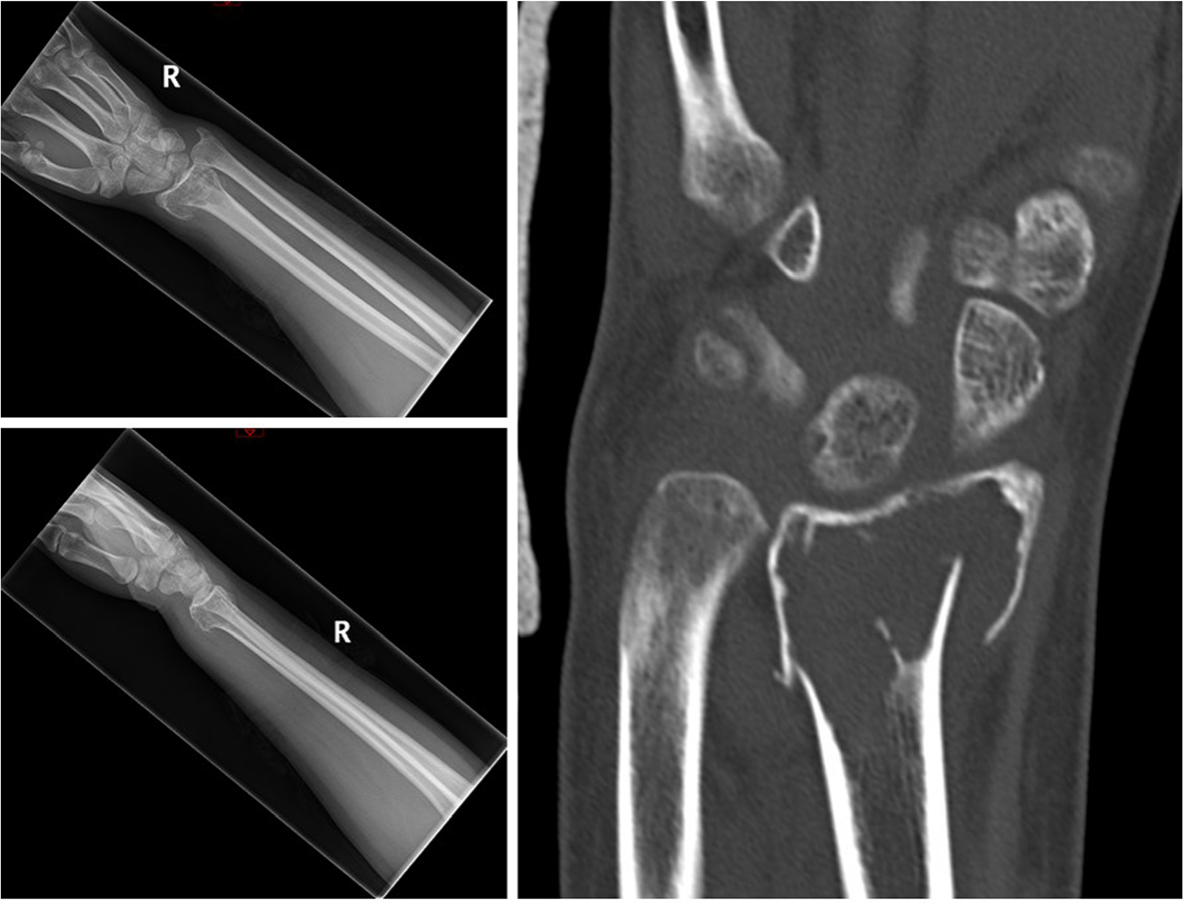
ap and lateral plain radiographs and coronal CT of initial imaging of right wrist
